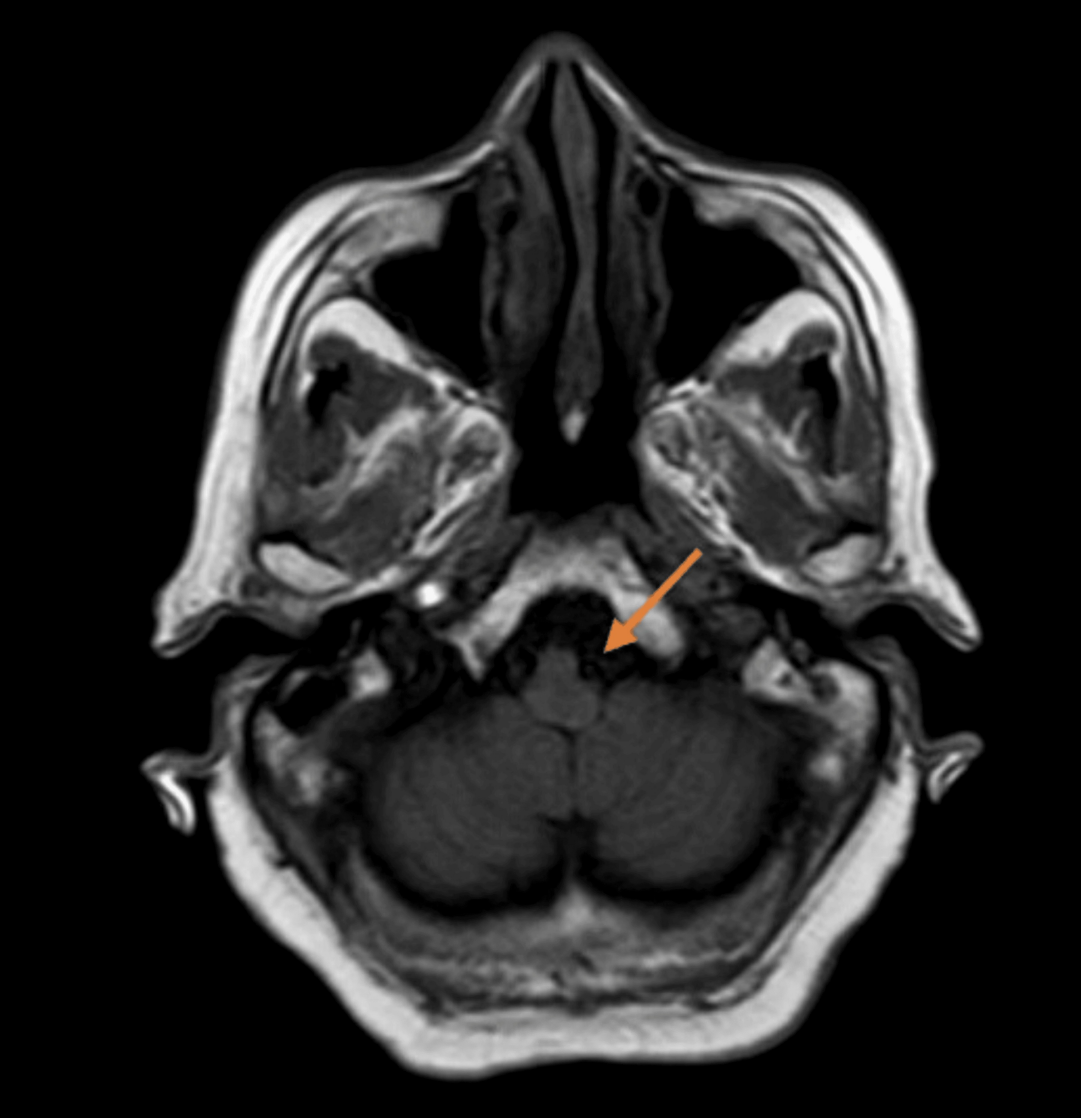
T1-weighted image of the brain-axial section showing ectatic left vertebral artery compressing the left anterolateral medulla T1WI, T1-weighted image
